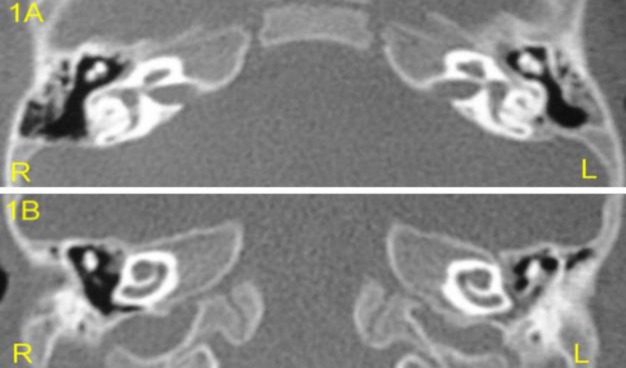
Pre-operative axial (A) and coronal (B) computed tomography scan showing patent basal turn of the cochlea on both sides
